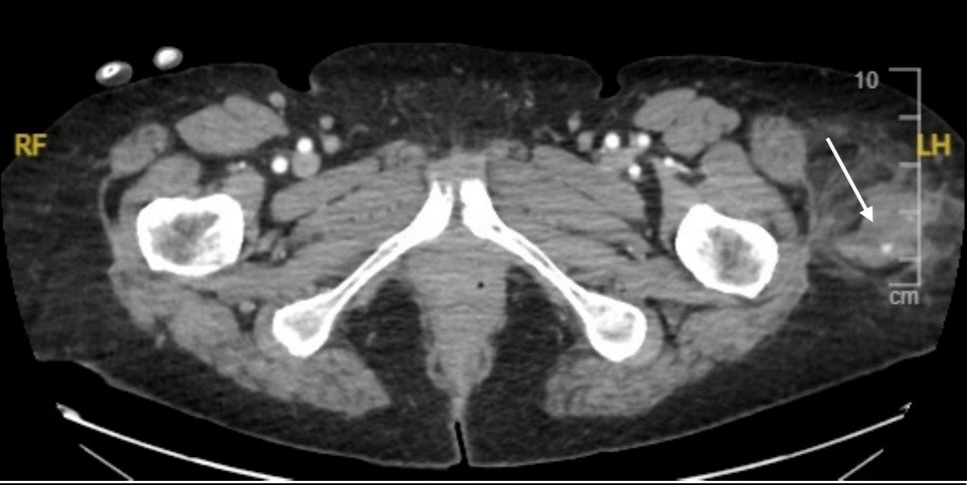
66-year-old female presenting to the emergency department after a fall from standing height. CT scan demonstrated a left lateral hip/upper thigh subcutaneous haematoma with contrast extravasation (arrow). The patient was successfully managed conservatively
